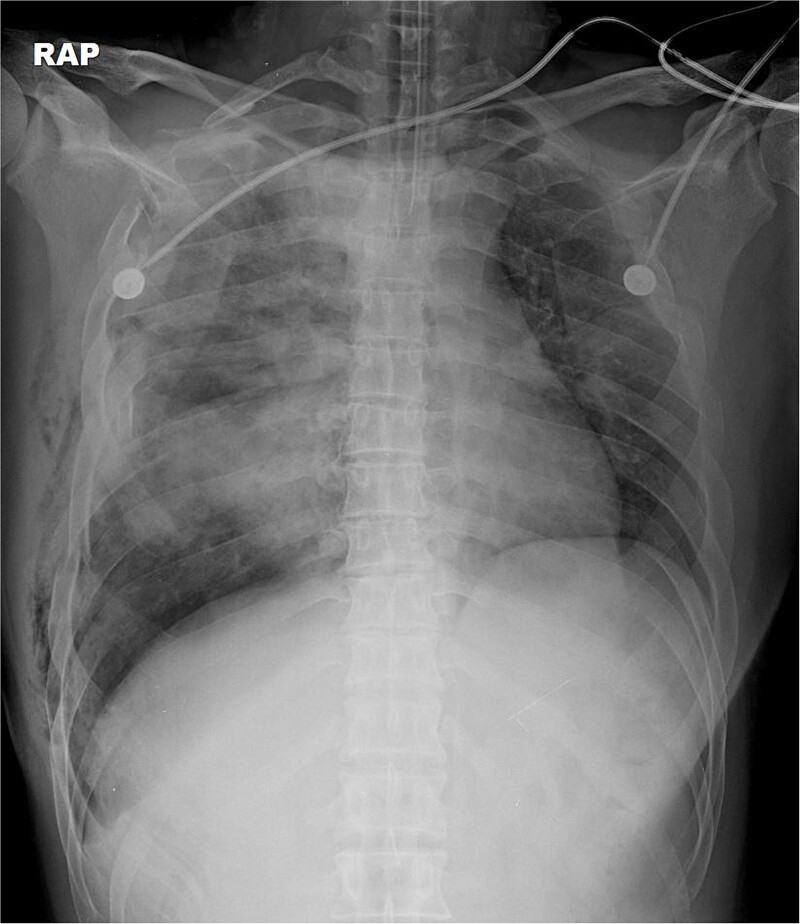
A simple radiograph of a 31-year-old man after injury.
